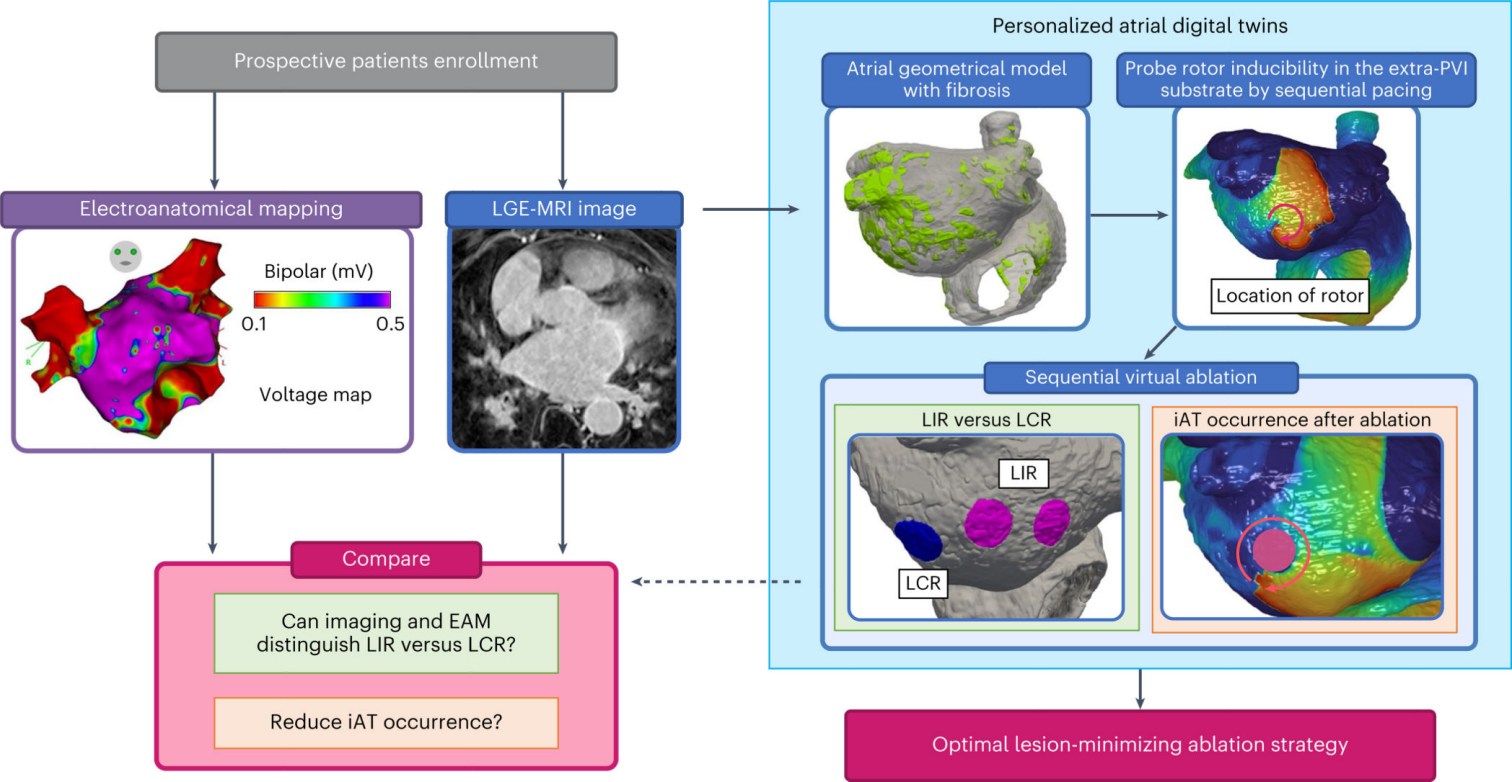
Overview of the combined prospective clinical and personalized DT study. In patients with PsAF enrolled prospectively, LGE-MRI images were acquired before the ablation procedure (blue panel, left). EAM maps were acquired during the procedure (purple panel, left). Right, light blue box, personalized digital twinning. A personalized atrial DT was constructed from LGE-MRI (top left image), and locations capable of sustaining rotors in the extra-PVI substrate were identified (top right image). LIRs, those capable of giving rise to sustained rotors and the preferred ablation targets for non-inducibility, are discerned from LCRs, those that lose their rotor-attracting capabilities when another rotor is eliminated (image within the green panel), and the likelihood of iAT occurrence at ablation locations is investigated (image within the orange panel). FD and FE values and EAM data were analyzed at these locations to uncover the features of LIRs and LCRs and of iAT-inducing locations (cranberry panel, left).
